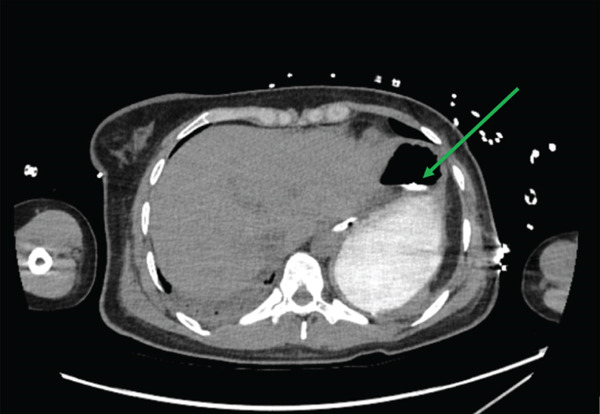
CT image of the hyperdense collection signifying a potential bezoar.
